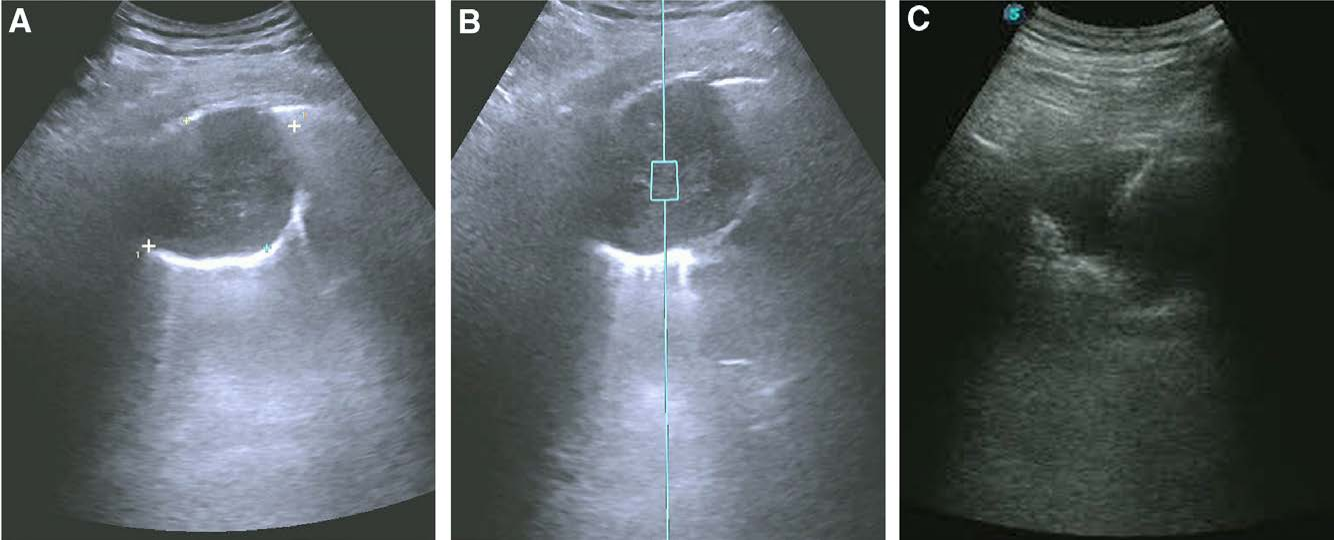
(A) Gray scale ultrasound indicates a hypoechoic mass under the right pleura, which is round in shape with unclear boundaries and uneven internal echoes. A “comet tail sign” can be seen behind the mass. (B) Shear wave elastography indicates an Emean of 7.9 kPa of the mass. (C) Ultrasound-guided mass puncture surgery.
